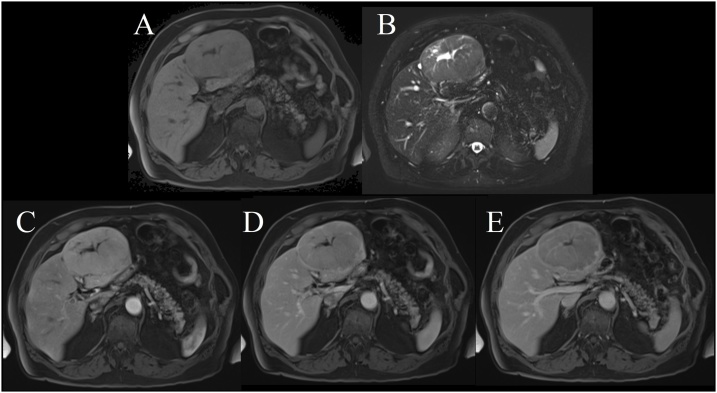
Primary hepatic ACC focal to segments 2 and 3. T1-weighted images shown as A, while T2-weighted images shown in B. The mass shows mild hypointensity, mild T2-weighted hyperintensity and restricted diffusion. Within it, a central stellate area of T1-weighted hypointensity and T2-weighted hyperintensity is visible. The internal central "scar" does not enhance. Within the mass, some radiating enhancing septae are visible. There is no fat nor lipid hyperexpression within the mass. The mass does exhibit mild diffuse enhancement in hepatic arterial phase (A – pre contrast; C – 0.5 min post contrast; D – 1 min post contrast) with slight portal venous phase washout and, on delayed imaging, a capsule (E – 5 min post contrast).
